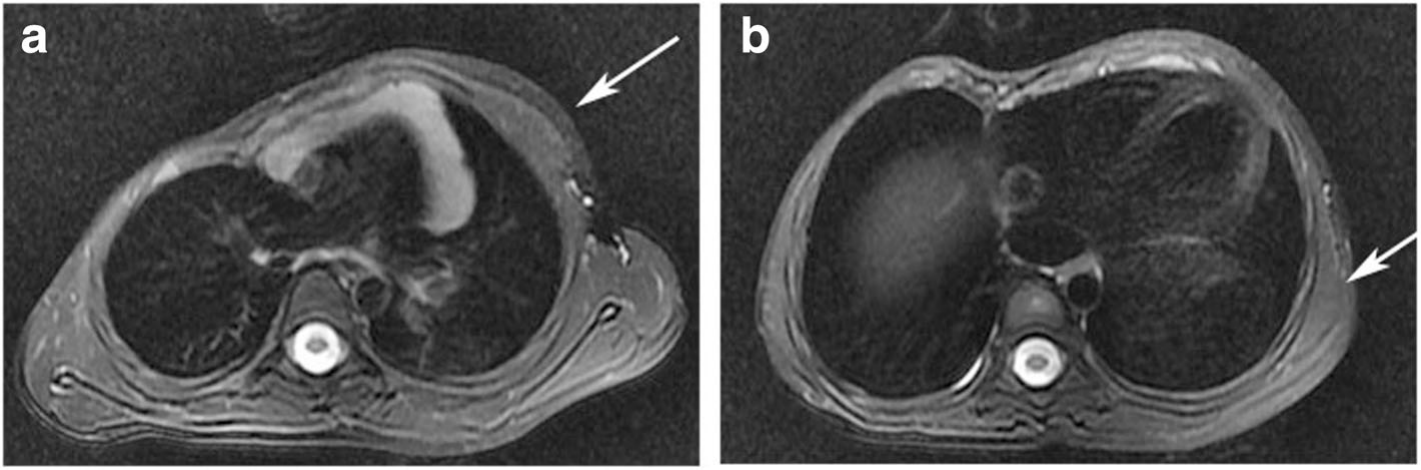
Chest magnetic resonance imaging. It revealed the pectoralis major and minor(a, arrowheads) and latissimus dorsi muscles(b, arrowheads) of the left side, and the absence of these muscles of the right side
